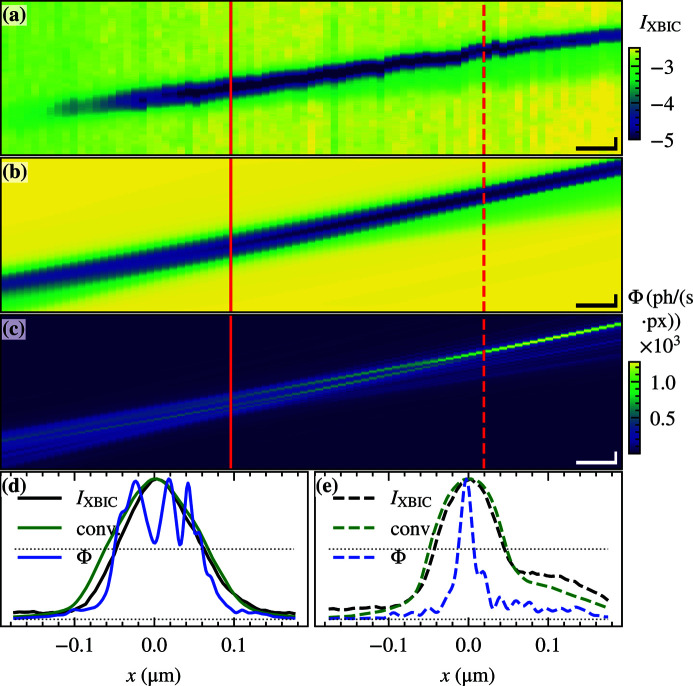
Comparison of the X-ray beam path using XBIC and ptychography. (*a*) XBIC measurement of an off-axis illuminated MZP with apertures. (*b*) Convolution of the ptychographically reconstructed probe with a nanowire profile. (*c*) The corresponding ptychographic reconstruction. The dashed line is the plane of focus; the solid line is the plane of measurement. (*d*) Line profile in the focus plane and (*e*) the measurement plane. Scalebars (*a*–*c*) vertical 100 nm, horizontal 10 µm, (*d*,*e*) 100 nm
